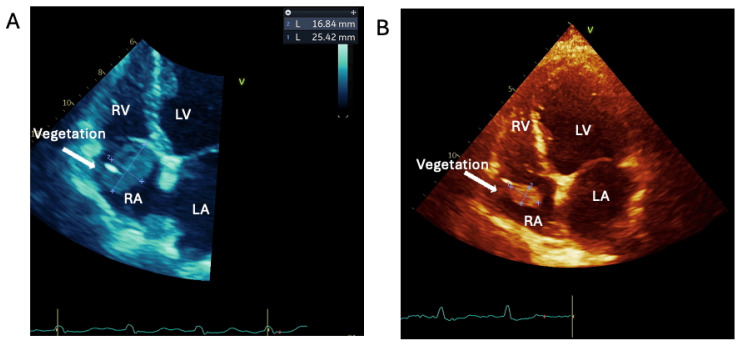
Apical 4-chamber view of the transthoracic echocardiography of case 1 with infected CIED in the right atrium with a large vegetation on the atrial site. (A): on admission, the size of the vegetation is 25 × 17 mm. (B): the vegetation 42 days after antibiotic IV and anticoagulation has reduced size to below 20 mm—this allowed the CIED to be safely extracted percutaneously. RV: right ventricle; LV: left ventricle; RA: right atrium; LA: left atrium.
